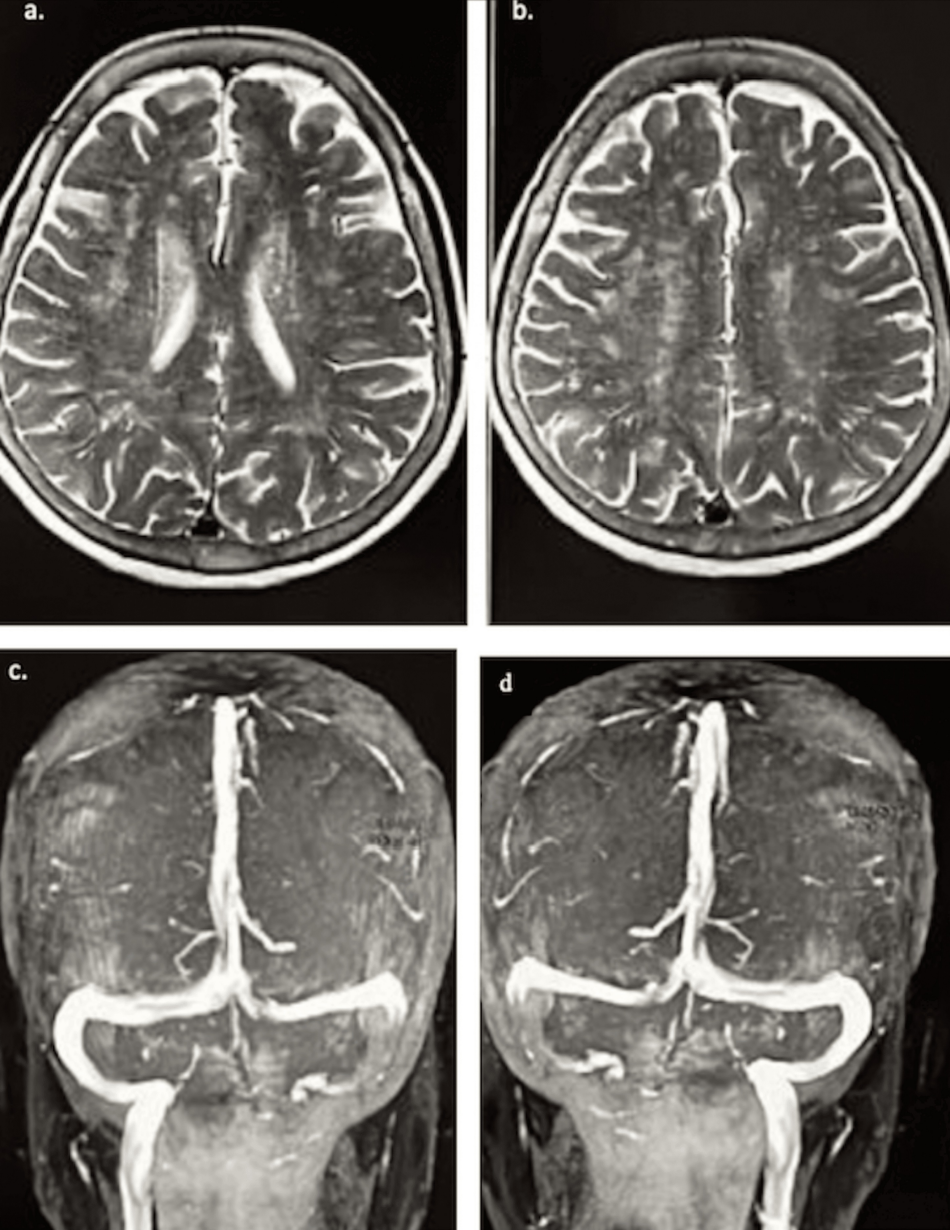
Magnetic resonance imaging (MRI) and magnetic resonance venogram (MRV) of the brain. (a, b) Axial plane section of MRI of the brain showing no abnormalities. (c, d) Coronal plane section of MRV of the brain showing no abnormalities.
